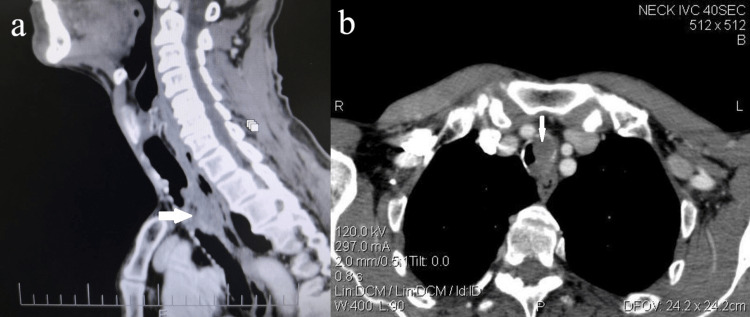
(a) Sagittal view of CT revealing the extension of the tumor (white arrow). (b) Axial view of CT revealing the extent of obstruction of a tracheal lumen (white arrow). CT: computed tomography
